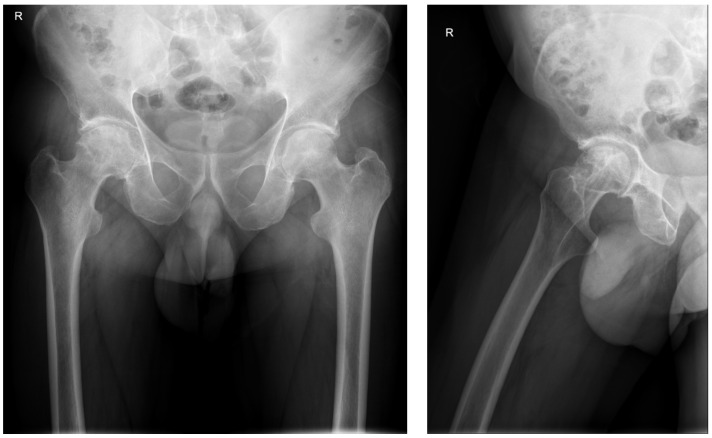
X-ray of the right hip on admission.
